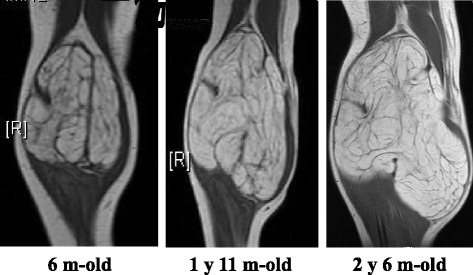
Magnetic resonance images showing the clinical course. The mass gradually increased in size from 6 months to 1 year and 6 months of age, after which it grew rapidly, eventually preventing the patient from walking. *m* month, *y* year
